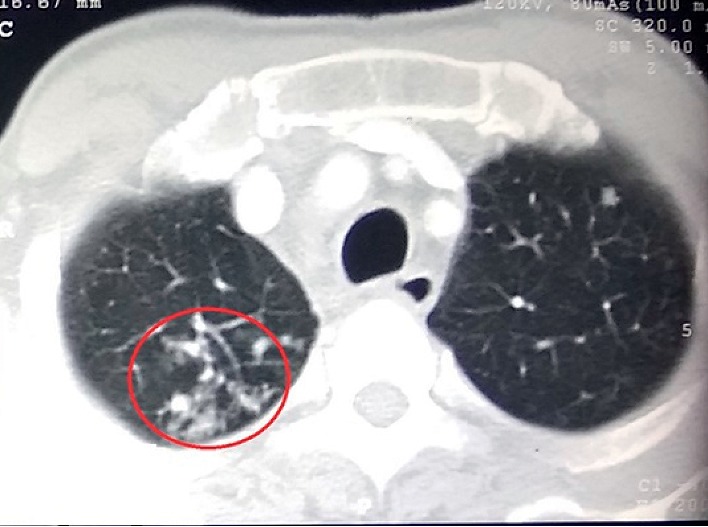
Contrast CT showing “tree-in-bud” appearance in the right upper lobe (circle) of the lung.
